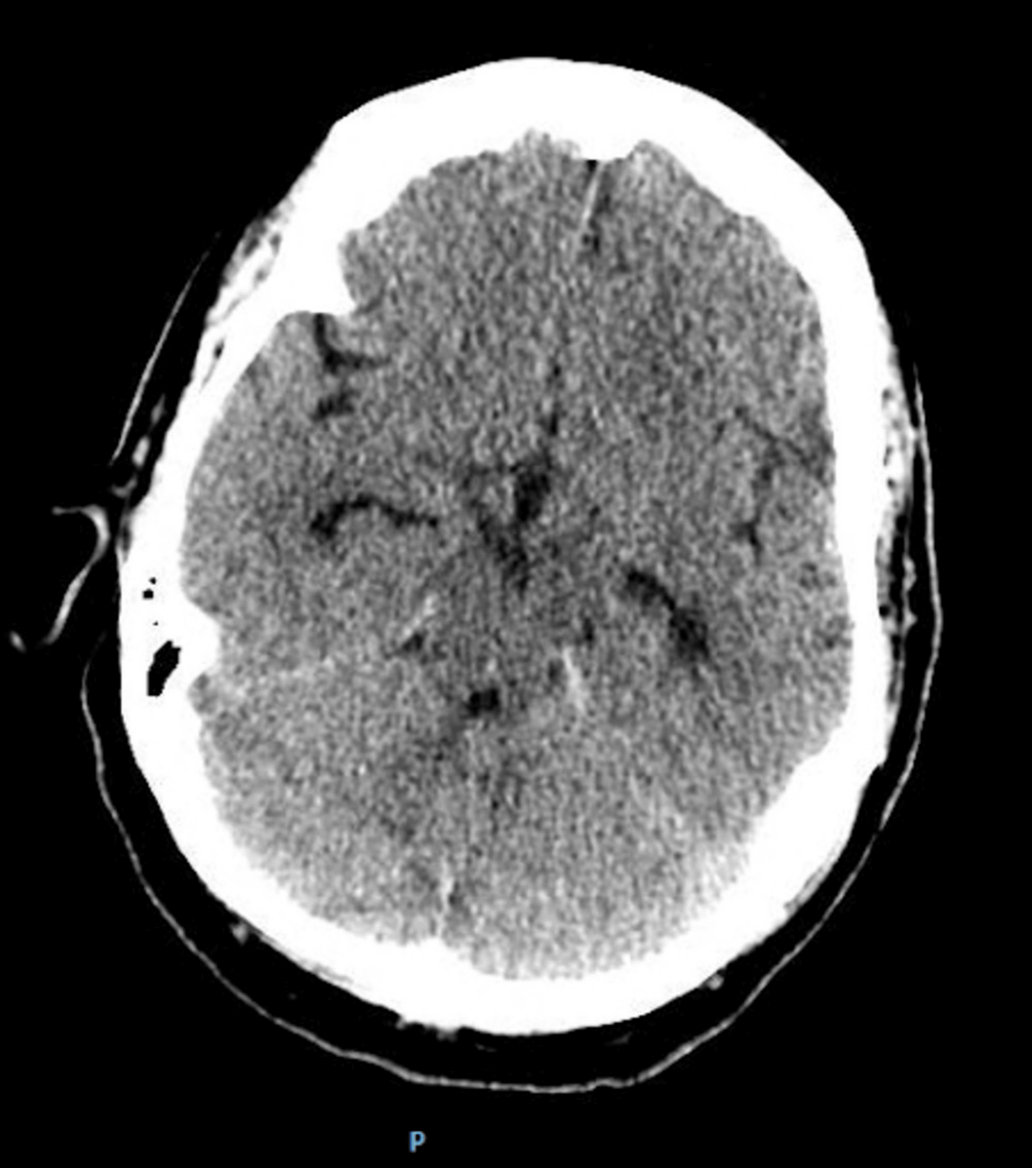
Non-contrast computed tomography (CT) scan of the head showed no acute intracranial abnormality.
